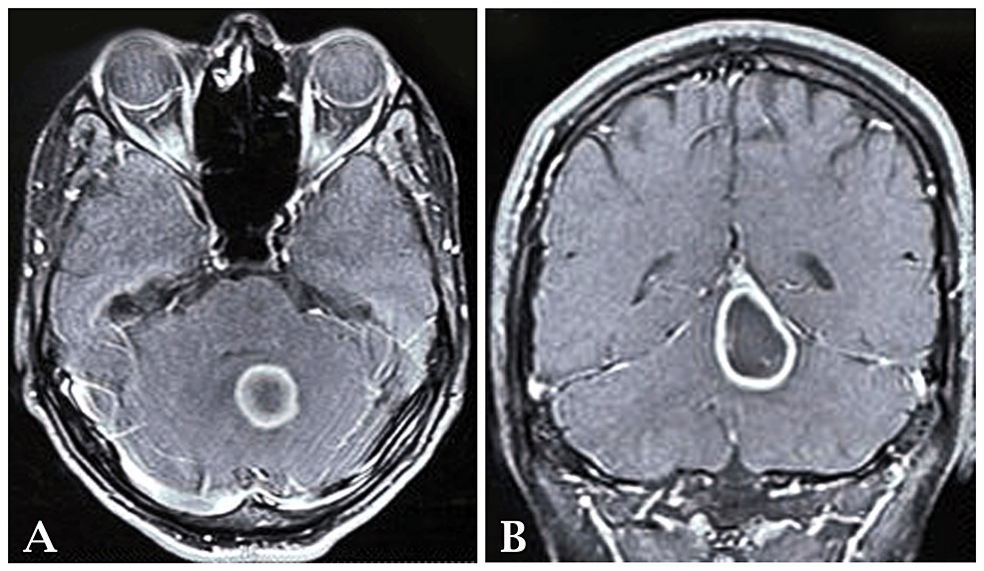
(A and B) The images show the presence of rounded to oval-shaped ring-enhancing lesions involving the left cerebellar vermis, displaying hypointense T1W1 and hyperintense T2W1 and FLAIR signals, with mild restricted diffusion on diffusion-weighted imaging (DWI), and perilesional vasogenic edema exerting mass effect on the fourth ventricle, indicating a likely abscess or neoplastic lesion. FLAIR: fluid-attenuated inversion recovery
